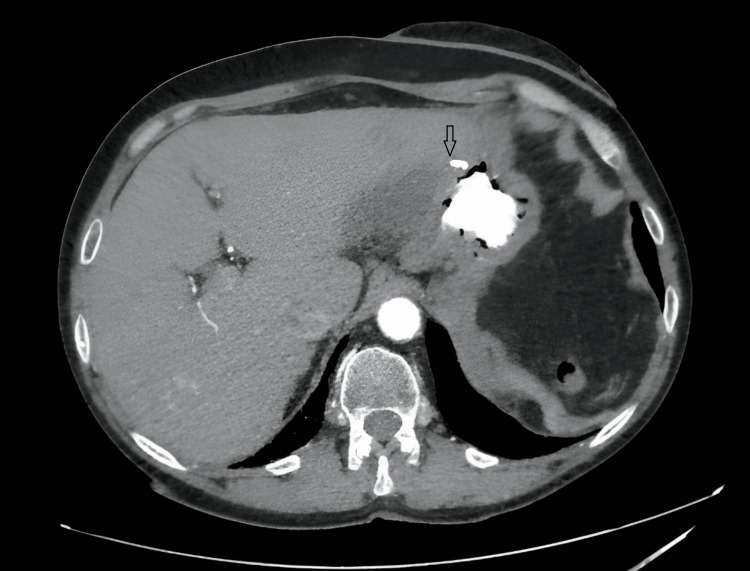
CT image obtained on the 5th postoperative day. In the early postoperative CT images, the staple line (arrow) in the anterior stomach is visible. No contrast material leakage, free fluid, or infectious content is observed.
